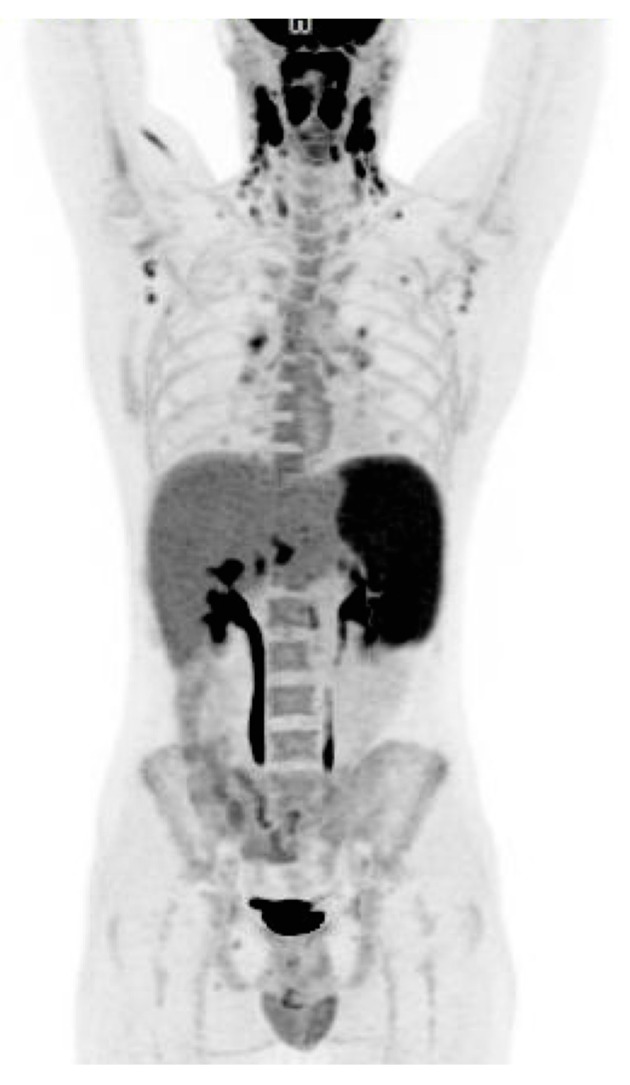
A 21-year-old man presented with 6 weeks of unexplained fever, pharyngitis, enlarged cervical neck lymph nodes, night sweats and severe fatigue. The patient was treated as an outpatient with antibiotics for acute tonsillitis twice without any improvement. He had persistent daily fever and a 6 kg unintentional weight loss. No significant past medical history. Epstein-Barr virus (EBV) and Cytomegalovirus (CMV) titers drawn one week after debut of symptoms were negative. Three months before presenting with symptoms the patient had been travelling in South East Asia for 3.5 months. He had not been sick during his travel abroad. Physical examination was significant for lymphadenopathy at the cervical neck. No splenomegaly was noted. At admission white blood cells was 13.0 × 10^9^/L (normal range: 3.5–8.8 × 10^9^/L) with 10.0 × 10^9^/L lymphocytes (normal range: 1.0–3.5 × 10^9^/L). Alanine aminotransferase (ALT) was elevated at 104 U/L (normal range: 10–70 U/L) and Lactate dehydrogenase (LDH) was elevated at 939 U/L (normal range: 105–205 U/L). Due to concern of malignant lymphoma an ^18^F-FDG PET/CT was performed (Figure 1). The scan revealed pathologically ^18^F-FDG uptake in enlarged lymph nodes in the bilateral regions of neck, axillas, lung hili, and in an enlarged spleen (18 cm). Findings were suspicious for malignant lymphoma. A lymph node biopsy was considered.
